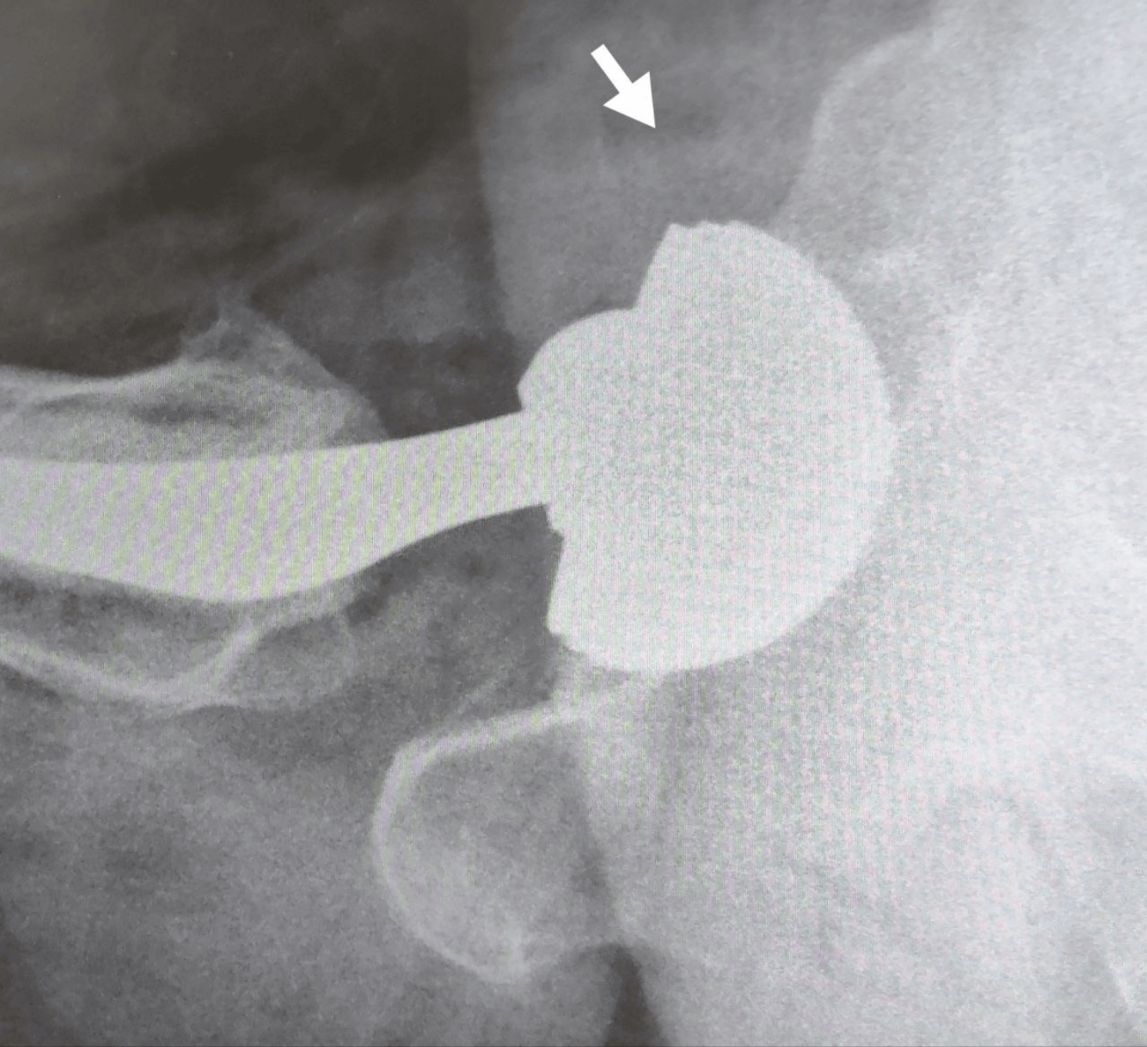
Cross-table radiograph with an arrow demonstrating anterior overhang of the acetabular component Image credit: Second author (consent obtained from the patient for use of the image in research).
